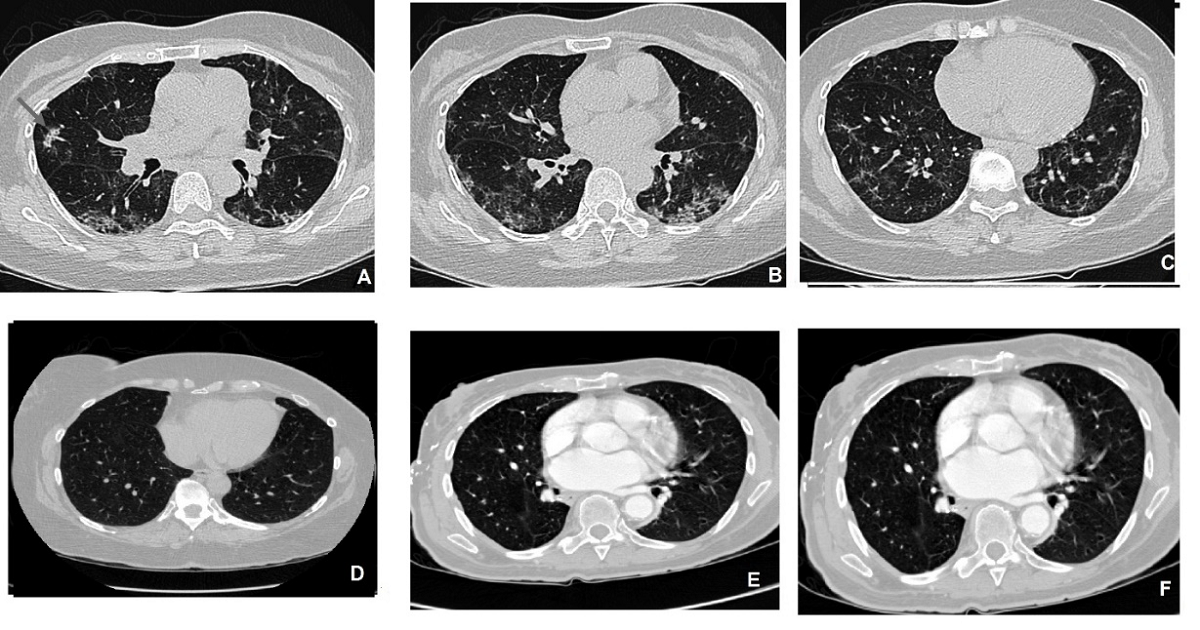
Axial computed tomography scan slices of the lung. (A, B, C) Non–COVID-19 cases including those of pneumonia and healthy individuals; (D, E, F) infected lungs of patients with COVID-19.
